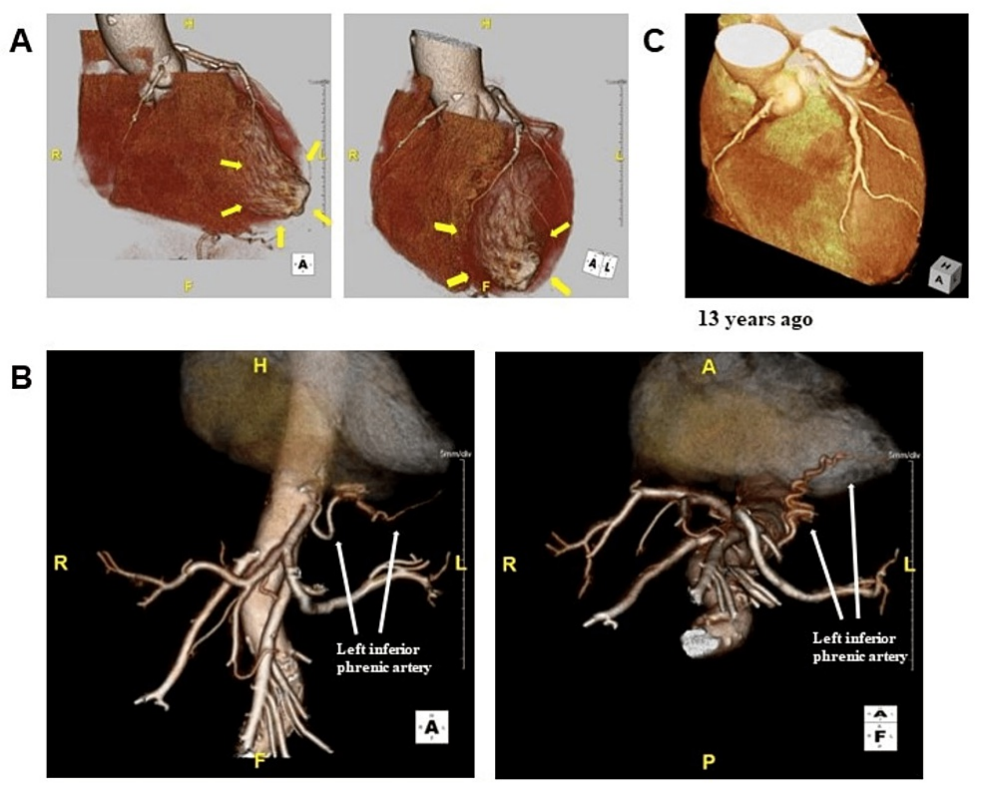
Computed tomography angiography. (A) No lesions in the coronary arteries consistent with coronary angiography. However, the apex with aneurysm appeared to be looked like an avascular field (arrows). (B) The left inferior phrenic artery, which branches directly from the descending aorta, was markedly dilated to nourish the apex of heart. (C) 13 years ago: the arrangement of vessels had not changed before the formation of the aneurysm.
